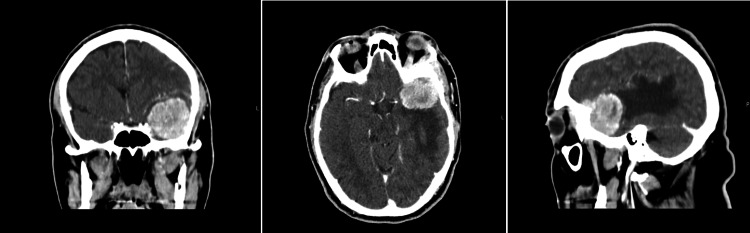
Coronal, cross-sectional, and sagittal views of the head CT scan sections showing a space-occupying lesion
